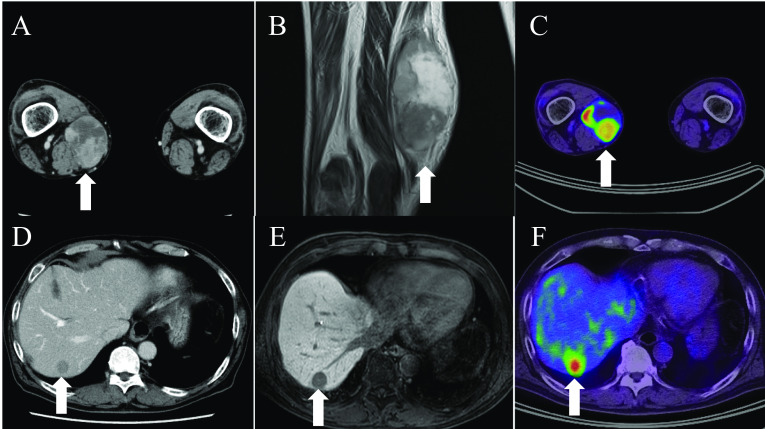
A Contrast-enhanced computed tomography (CT) revealing a mass with a heterogeneous contrast effect in the right thigh. B T2-weighted magnetic resonance imaging (MRI) showing a solid tumor with high intensity in the right medial thigh, which is suggestive of soft-tissue sarcoma. C 18F-fluorodeoxyglucose-positron emission tomography CT indicating fluorodeoxyglucose accumulation with a maximal standardized uptake value of 12.9 in the right thigh. D Contrast-enhanced CT revealing a liver tumor with a low contrast effect in the liver S7. E Ethoxybenzyl-diethylenetriamine pentaacetic acid (EOB) MRI indicating defective EOB uptake in liver S7. F 18F-fluorodeoxyglucose-positron emission tomography CT showing fluorodeoxyglucose accumulation with a maximal standardized uptake value of 12.3 in liver S7
